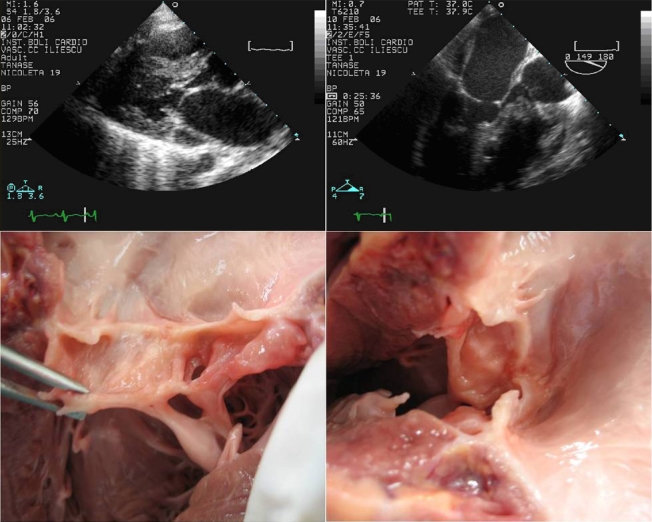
Transthoracic echocardiography: parasternal
